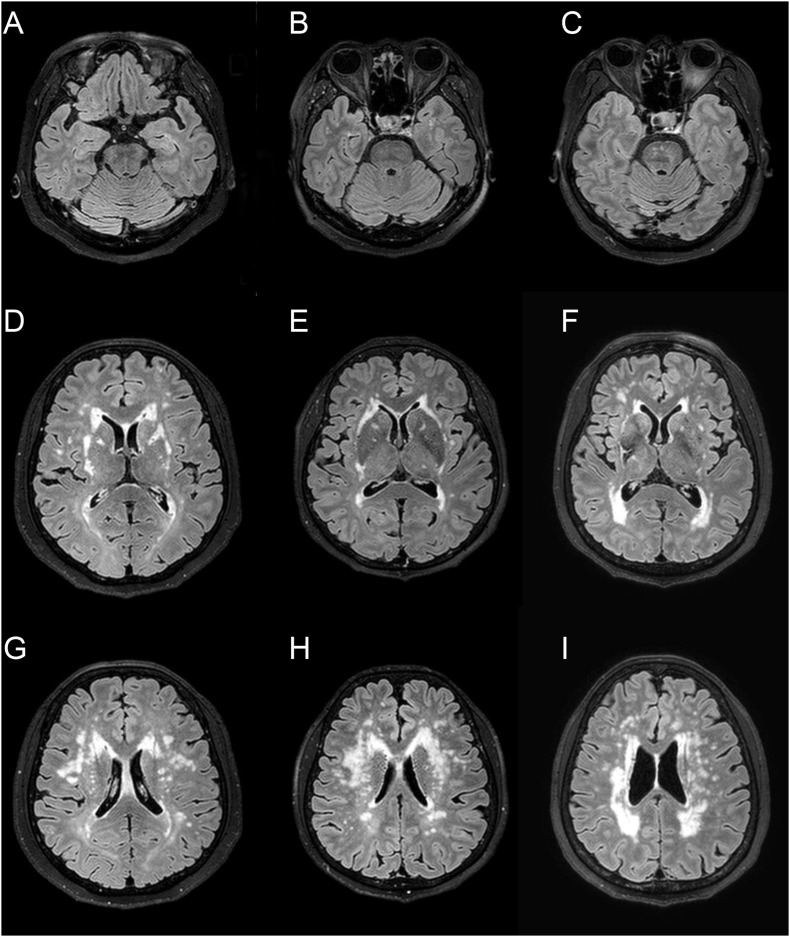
Representative fluid-attenuated inversion recovery MRI of three CADASIL patients with different APOE genotype. Patient 1 (A,D,G) is a 66-year-old female with APOE ε3/ε3 [normalized volume of white-matter hyperintensities (nWMH) = 0.0048]. Patient 2 (B,E,H) is a 67-year-old female with APOE ε2/ε3 (nWMH = 0.0051). Patient 3 (C,F,I) is a 66-year-old female with APOE ε3/ε4 (nWMH = 0.0049). All patients had p.Arg544Cys mutation.
